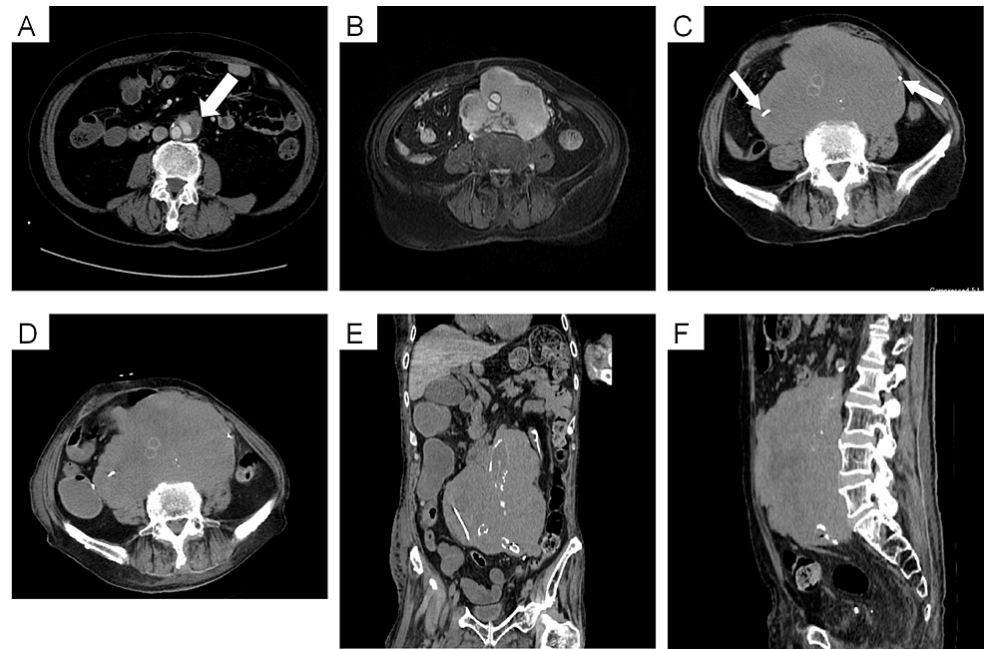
Imaging findings over time (A) Contrast-enhanced computed tomography (CECT) image acquired 22 months before death (i.e., 37 months postoperatively). The arrow shows a seroma-like, low-density area around the Y-shaped graft in the abdominal aorta and common iliac arteries. (B) A contrast-enhanced, T1-weighted magnetic resonance image obtained 15 months before death (i.e., 44 months postoperatively) shows that the mass increased to approximately 10 × 9 cm. (C) A simple CT scan obtained five months before death (i.e., 54 months postoperatively) shows further enlargement of the mass. The arrows indicate the site of ureteral stent replacement. (D, E, and F) Simple CT scans obtained 59 months postoperatively show the horizontal, coronary, and sagittal sections, respectively. A mass, measuring approximately 17 × 16 × 12 cm in each section, is noted in the retroperitoneum.
